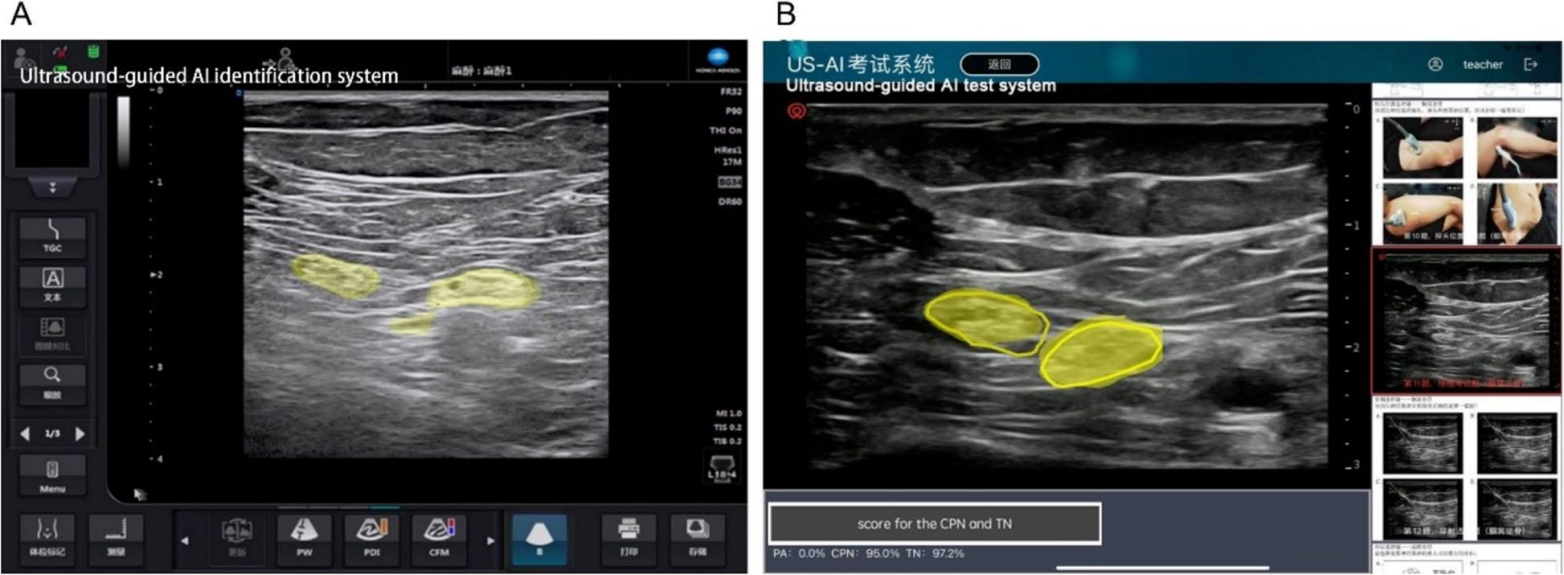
Artificial intelligence-assisted identification system for ultrasound-guided sciatic nerve block training. A AI-assisted identification system used to train residents to perform ultrasound-guided sciatic nerve block via the popliteal approach. The yellow areas represent the TN and CPN. B AI-assisted test system to give feedback to residents. The test score was calculated by determining the extent of the intersection between the nerve area marked by the trainee (yellow area) and that identified by AI (area inside the yellow line)
